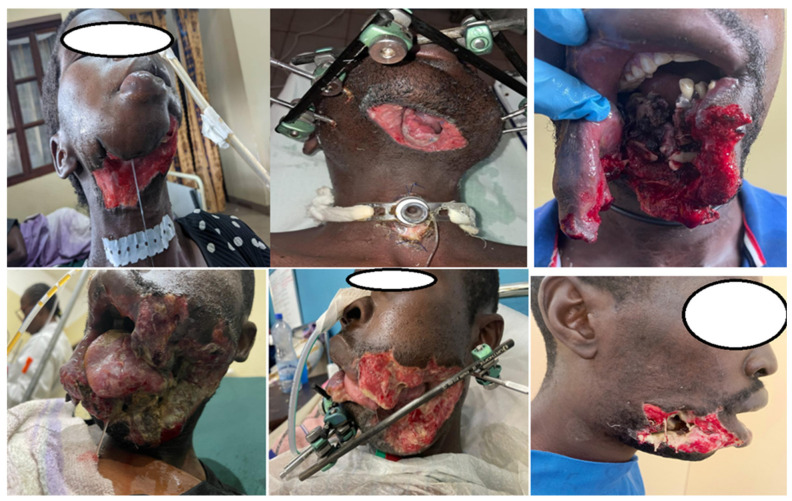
Clinical and radiographic aspects of a high-velocity gunshot injury causing a severely comminuted mandibular fracture with associated soft-tissue destruction and clinical evidence of infection at presentation.
